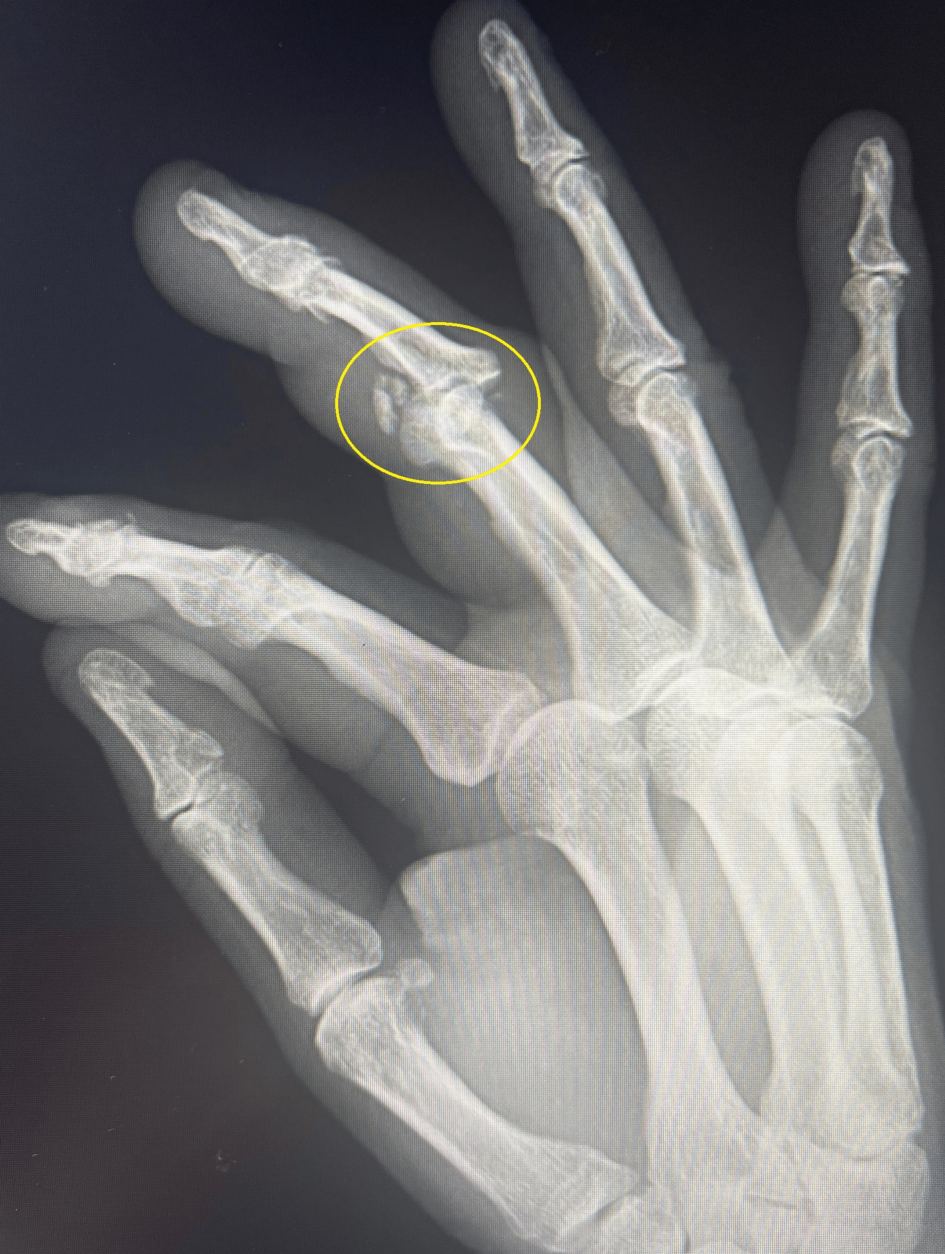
Comminuted pilon fracture of the PIP joint (X-ray in Robert's projection) PIP: proximal interphalangeal
